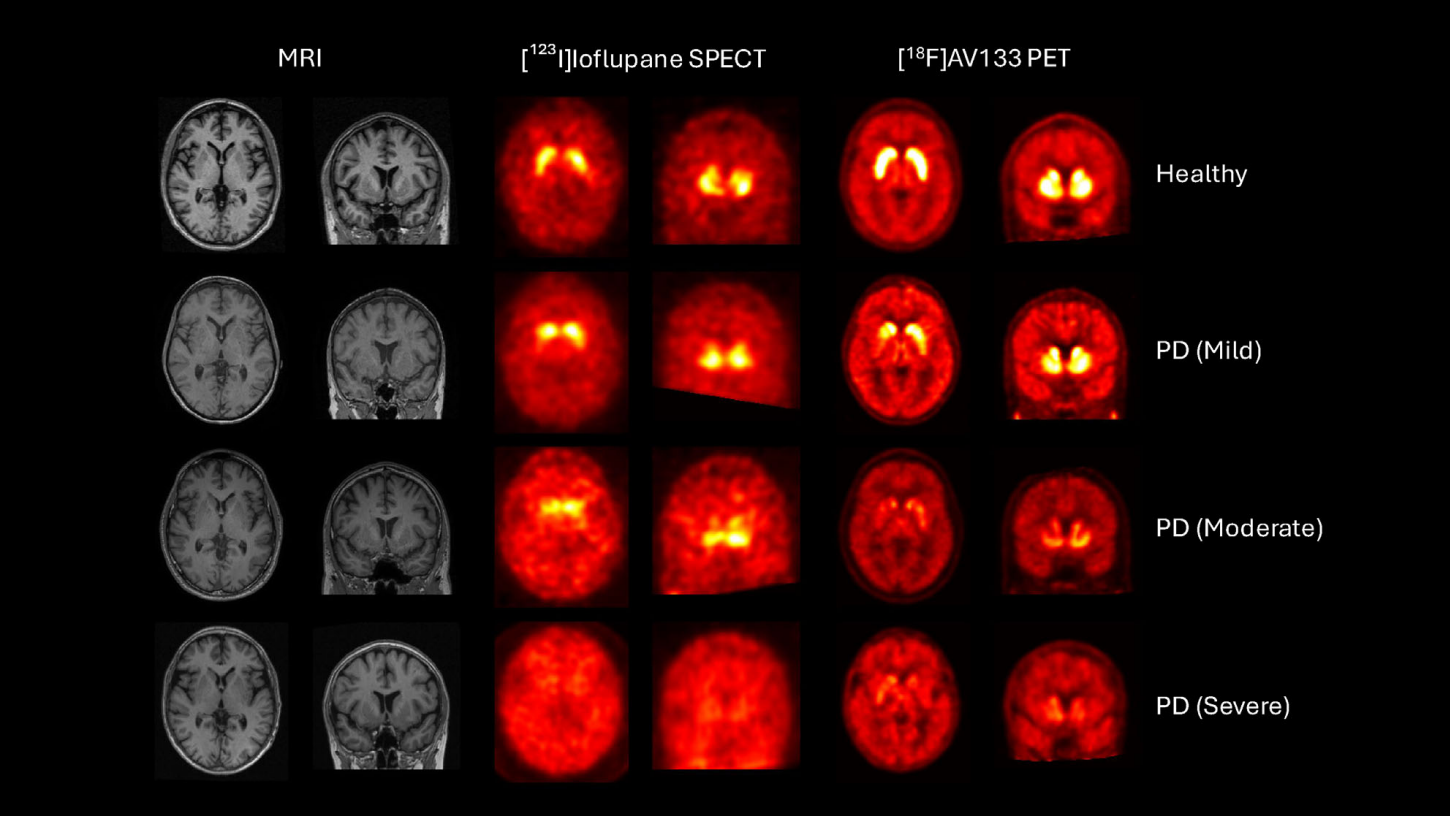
[^123^I]ioflupane single photon emission computed tomography (SPECT) (dopamine transporter [DAT]), [^18^F]AV133 positron emission tomography (PET) (VMAT2) and associated structural T1‐magnetic resonance imaging (MRI) images for a healthy subject, mild, moderate, and severe Parkinson's disease (PD) subjects. [Color figure can be viewed at www.annalsofneurology.org]
